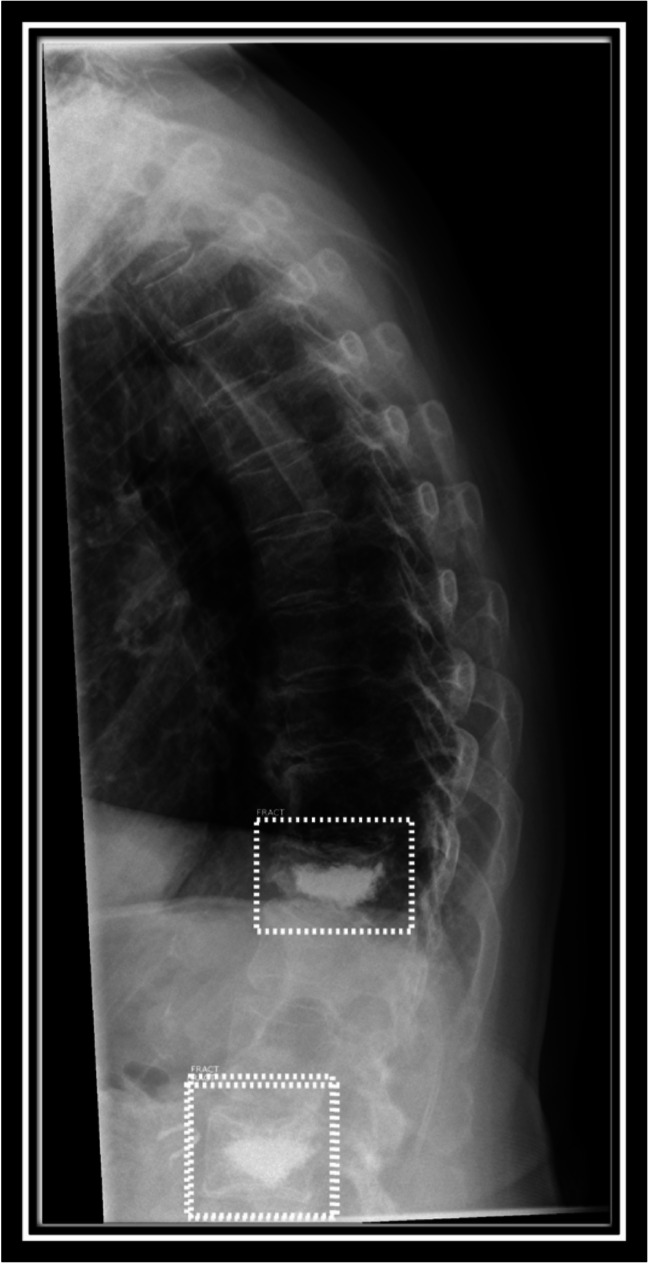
Lateral radiographs of the lumbar spine with markings by the AI software. Two dashed-line bounding boxes are erroneously placed around the L2 vertebrae as a potential fracture, where kyphoplasty was previously performed. All bounding boxes represent a true-positive, older fracture
